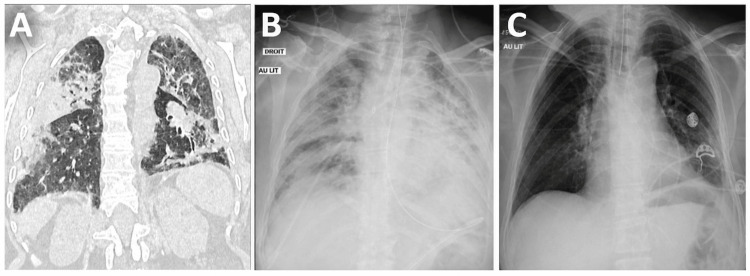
Imaging findings and disease evolution (A) Chest computed tomography (CT) showing bilateral multilobar consolidations with ground-glass opacities, predominantly in the left lung. (B) Chest radiograph demonstrating extensive bilateral alveolar opacities consistent with acute respiratory distress syndrome (ARDS). (C) Follow-up chest radiograph showing marked regression of pulmonary infiltrates after corticosteroid therapy and discontinuation of letrozole.
